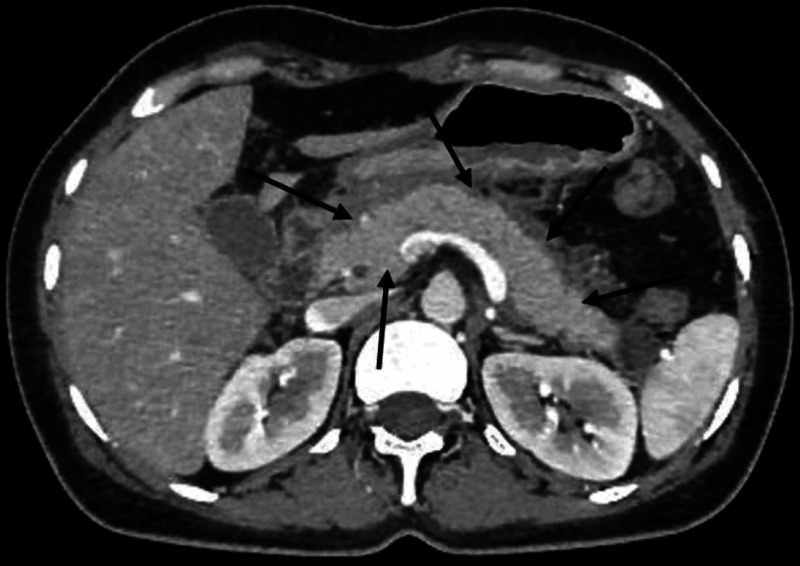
Transverse section of CT abdomen showing diffuse enlargement of pancreas with ill-defined borders and surrounding peripancreatic fluid.
